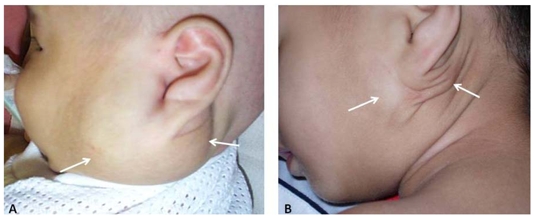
(A) A large disfiguring lymphangioma over the angle of left mandible (arrows) in a 3-month-old baby (Case 9). This patient’s ultrasound image is shown in Figure 2. (B) One year later, the appearance after three injections of bleomycin, significant reduction in size was observed. A small swelling (arrows) was noticeable in the pre- and post-auricular area.
